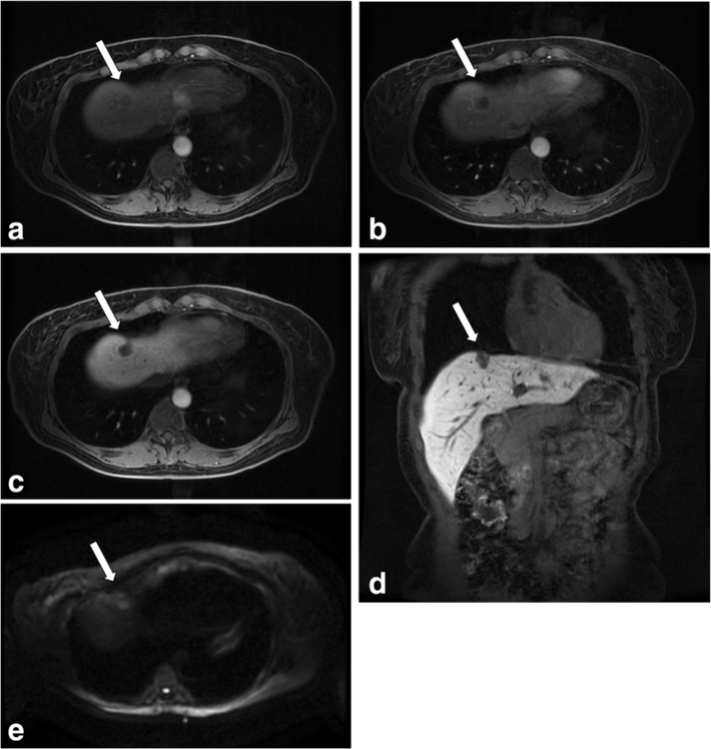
Axial slices of liver acquisition with volume acceleration flex on dynamic MRI in pre-contrast phase (a), arterial phase (b) and the hepatobiliary phase (c). Coronal slice on enhanced MRI in the hepatobiliary phase (d). The lesion (*arrows*) gave a low intensity signal in all phases and a high intensity signal in DWI (e)
